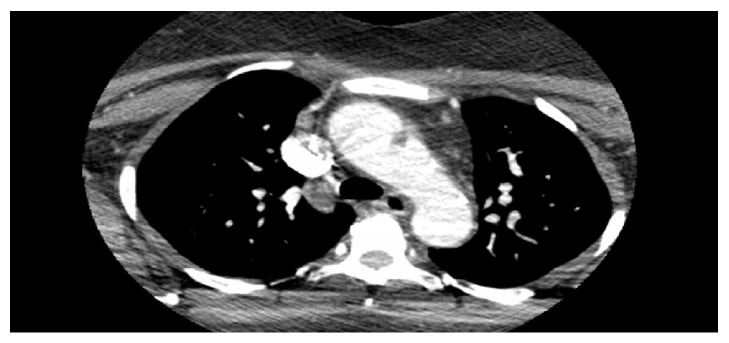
CT Angiogram of head and neck showing a 1.3 cm filling defect in the proximal aortic arch abutting the lateral wall of the aortic arch, consistent with thrombus.* Abbreviation.* CT: computed tomography.
